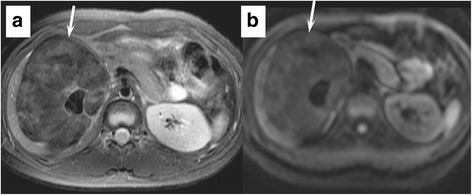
Abdominal magnetic resonance imaging. a Fat-suppressed T2-weighted fast spin-echo image (TR/TE, 1600/80 ms) shows a heterogeneously hypointense lesion, suggestive of abundant fibrosis (arrow). b Diffusion-weighted echo-planar image (TR/TE, 2291/47 ms) shows heterogeneous hypointensity without strong diffusion restriction (ADC value = 1.39 × 10^−3^ mm^2^/s) (arrow)
